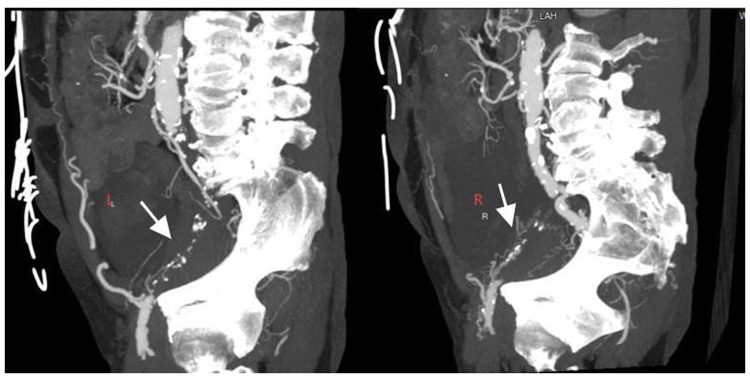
CTA revealing bilateral external iliac artery occlusion (white arrows).
